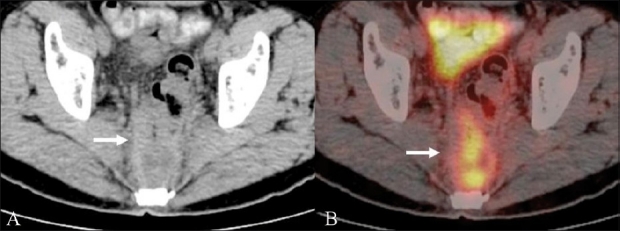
A 57-year-old man with a history of abdominoperineal resection (APR) for rectal cancer presented with symptoms of pelvic pain and underwent a restaging FDG PET / CT. Axial unenhanced CT scan (A) shows a heterogeneous soft tissue mass in the presacral region (arrow). Fusion PET / CT image (B) reveals increased FDG uptake within the soft tissue (arrow), suggestive of local recurrence
